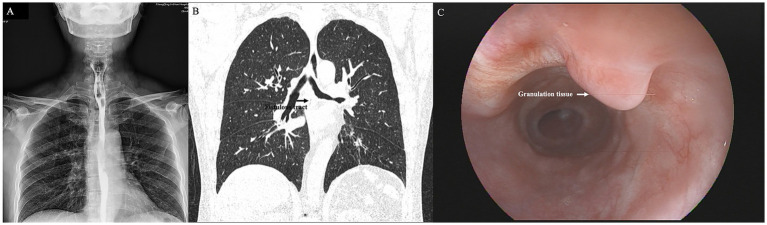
Postoperative follow-up at 10 months. (A) Esophagography showing no contrast extravasation. (B) Coronal thoracic computed tomography demonstrating no persistent communication between the esophagus and left main bronchus, with marked reduction in the residual fistulous tract (black arrow) compared with prior imaging. (C) Esophagoscopy revealing granulation tissue (white arrow) at the previous fistula site (28 cm from the incisors), surrounded by white scarring, with no identifiable fistula orifice on repeated inspection.
